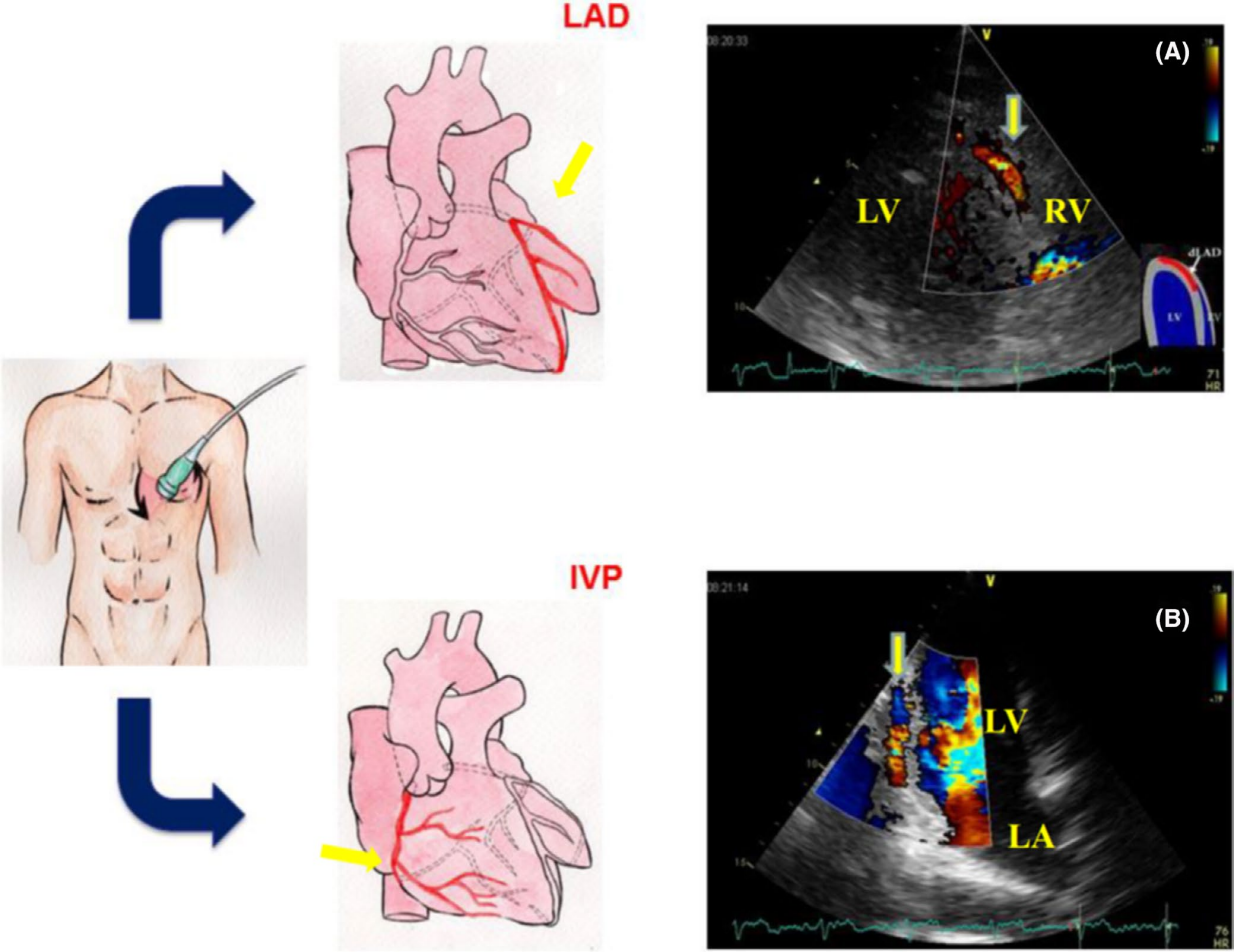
Color Doppler images of the distal left anterior descending coronary artery (LAD) and interventricular posterior coronary artery (IVP). The modified apical 4‐chamber (A) and 2‐chamber (B) positions, with cranial angulation of the transducer and a Nyquist limit of 20‐67 cm/s, allow optimal identification of the diastolic coronary flow. LV = left ventricle; RV = right ventricle; LA = left atrium
